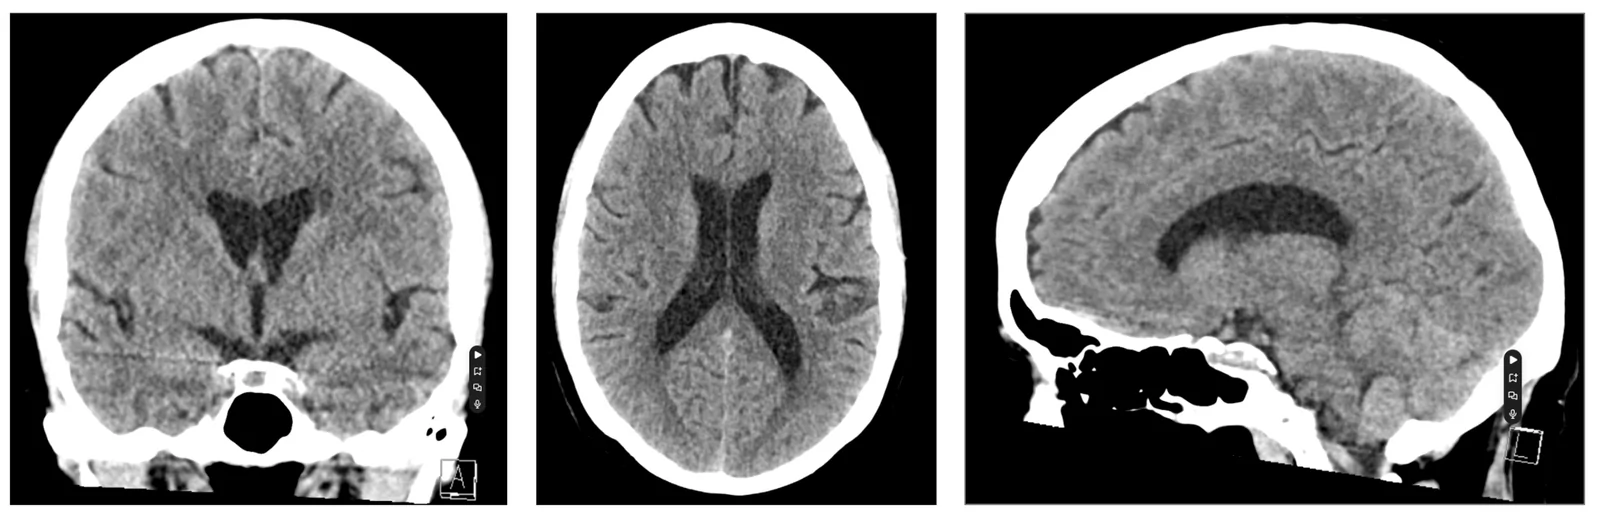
Non-contrast computed tomography images of the head obtained during the pre-electroconvulsive therapy evaluation. Representative (A) coronal, (B) axial, and (C) sagittal images respectively are shown. The imaging revealed no acute intracranial findings.
